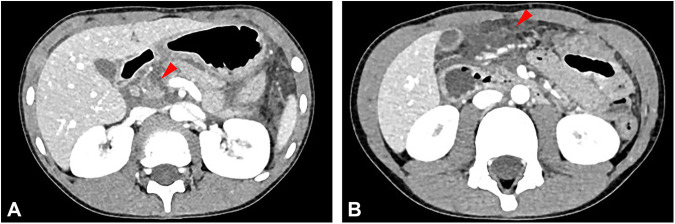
(Case 5): CT shows discontinuity of the pancreatic neck (a, red arrowhead), heterogeneous parenchymal density, blurred peripancreatic fat planes, and associated peripancreatic fluid collection (b, red arrowhead).
